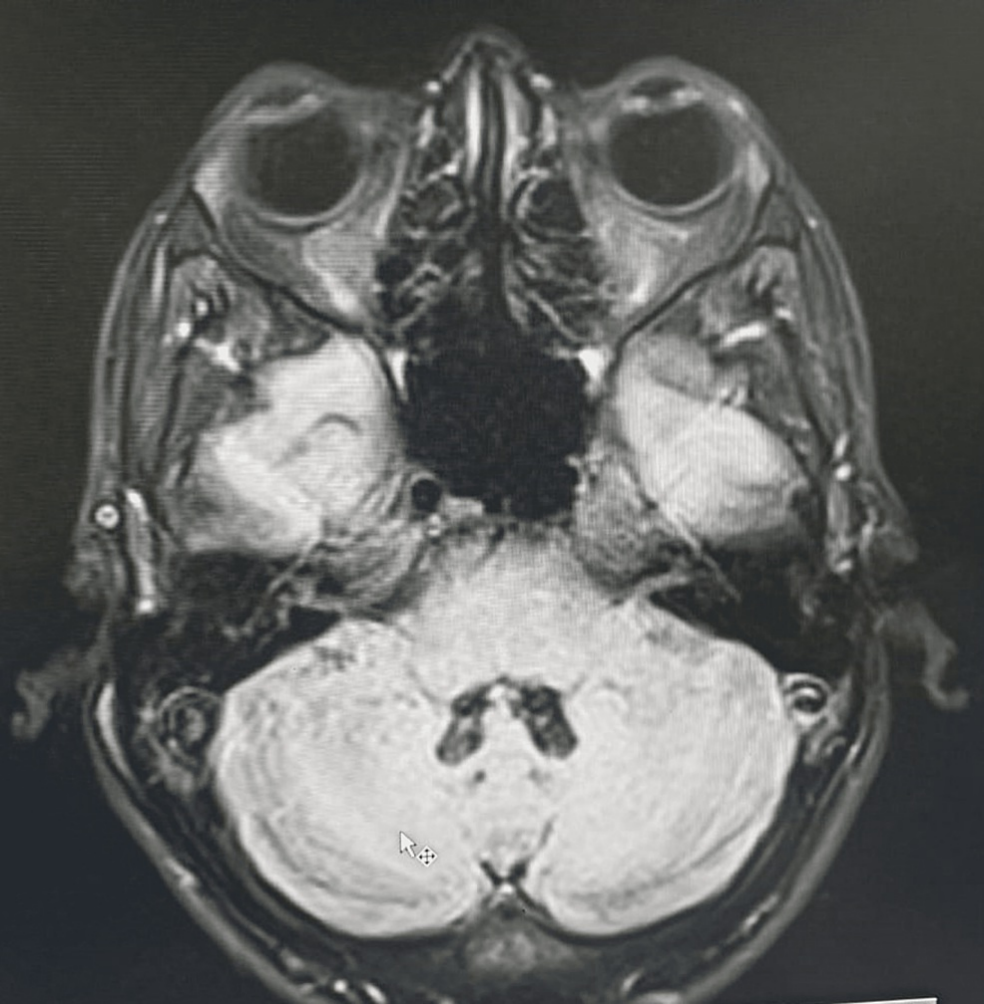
MRI brain findings in case 2 MRI brain FLAIR axial section showed normal bilateral dentate nucleus with no cerebellar atrophy (arrow)
